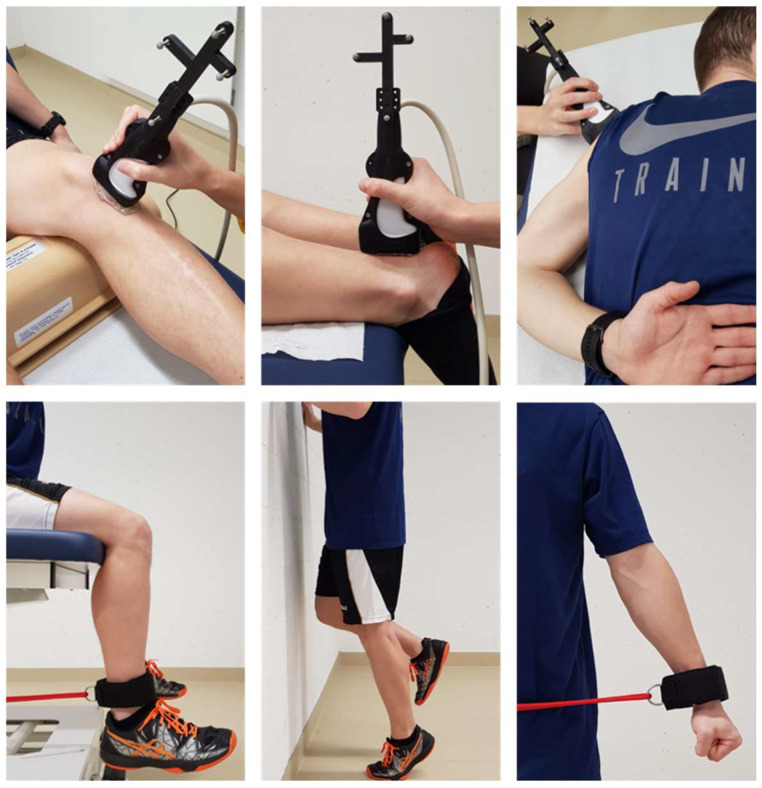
Top row: Subject position during ultrasound examination of the patellar, Achilles, and supraspinatus tendon, respectively. Bottom row: experimental setup used to induce tendon loading stimulus for either of the three tendons assessed.
